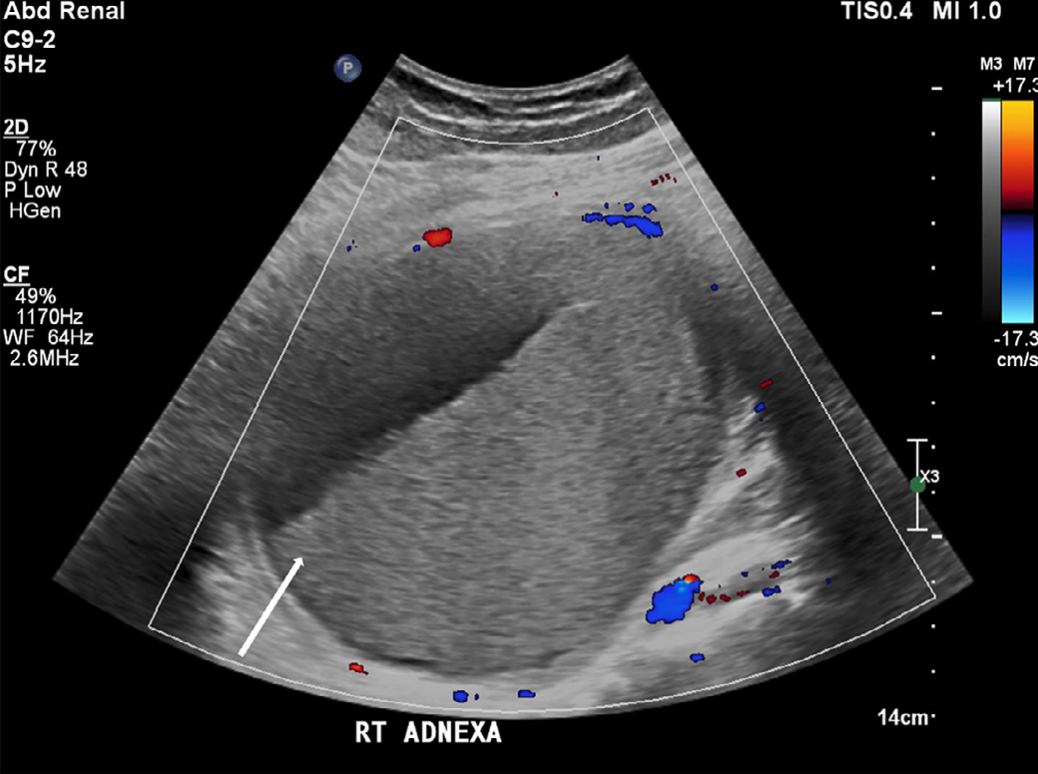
Transabdominal ultrasound of the pelvis with color flow with mildly increased peripheral vascularity. Again seen is fluid fluid layer within the endometrioma (white arrow).
